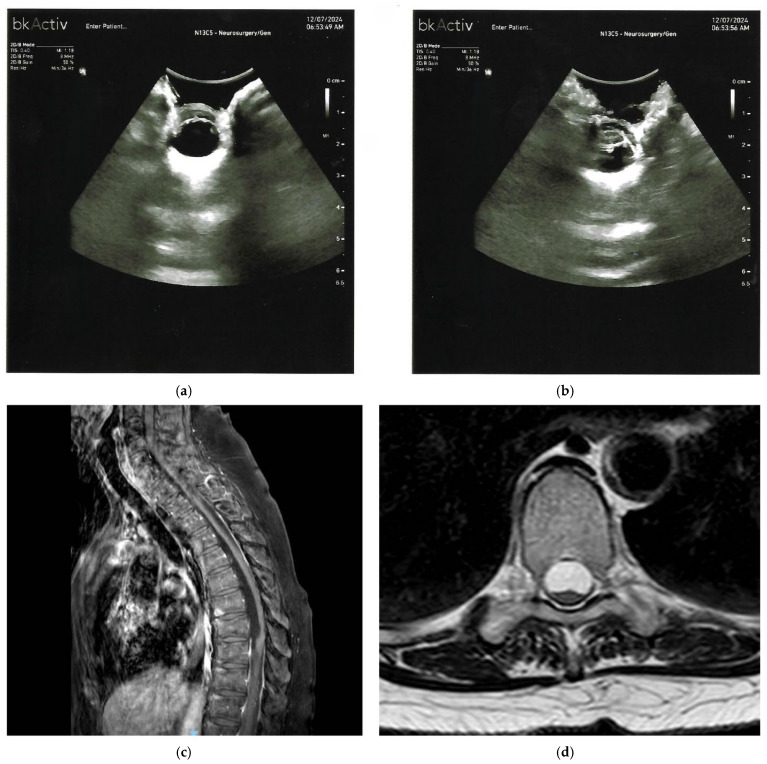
(a,b) Pre-durotomy intraoperative ultrasound demonstrates a predominantly anechoic/hypoechoic subdural collection immediately beneath the echogenic dura (arrow in (a) indicates echogenic membrane), bounded by a continuous echogenic membrane and containing fine internal echoes/septations (labeled ‘septations’ in (b)), consistent with a chronic hematoma and explaining the cord compression. The hypoechoic collection on IOUS (a,b) correlates with the elongated dorsal subdural collection on MRI (c). (c) Sagittal MRI shows an elongated dorsal subdural collection along the thoracic canal with mass effect and ventral displacement of the spinal cord (arrow indicates displacement). (d) Post-evacuation axial T2-weighted MRI showing re-expansion of the cord with restoration of the dorsal CSF space and near-complete resolution of the posterior subdural collection.
